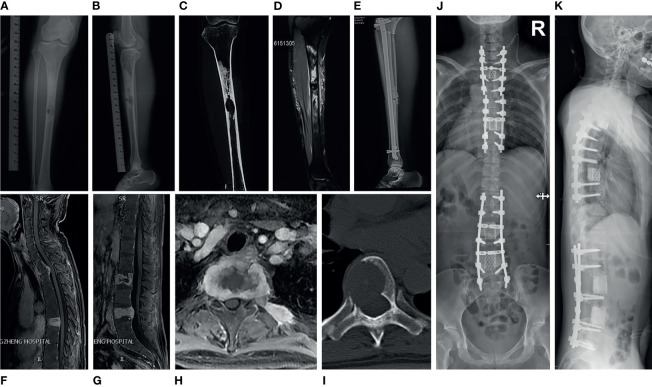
Medical imaging of adamantinoma in case 1. (A, B) Anterior and lateral X-ray of the left tibia showed that there were two osteolytic well-circumscribed lesions in the tibial diaphysis, surrounded by a large area of sclerosis. The larger lesion was located in the medullary cavity, the smaller lesion was inside the cortex. (C) CT of the left tibia demonstrated an well-demarcated osteolytic lesion in the middle of the diaphysis with surrounding large areas of dense sclerotic bone. (D) MRI of the left tibia lesion revealed intense heterogeneous post-contrast enhancement with a central cystic non-enhancing area. (E) Lateral radiograph of the post-operative left tibia showed tibial osseous graft reconstruction with placement of an intramedullary rod and interlocking screws. (F–H) MRI of spine showed irregular osteolytic destruction in T2, T7, L2, L4 vertebral body and posterior elements, accompanied by the formation of paravertebral soft tissue masses, which demonstrated heterogeneous post-contrast enhancement. (I) CT of the thoracic spine showed a round osteolytic destruction with a clear demarcation, which extend into the spinal canal and cause spinal cord compression. (J, K) Postoperative X-ray of the spine showed the patient underwent spondylectomy and reconstruction for three times to resect multiple segments tumors.
